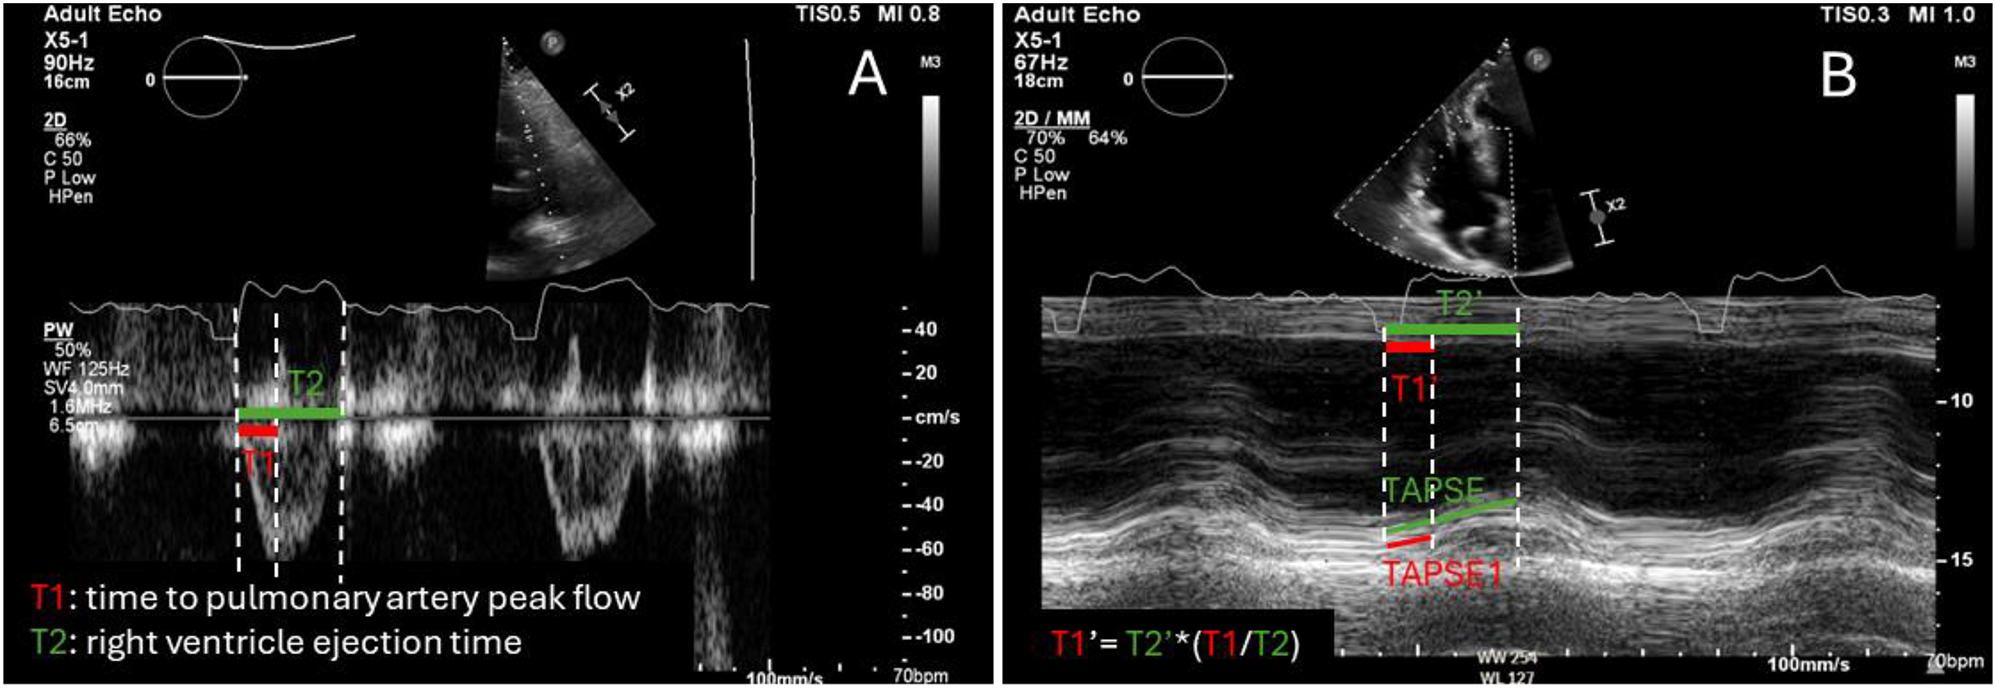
Measurement of first-phase TAPSE (TAPSE1). TAPSE1 was determined on echocardiogram by initially calculating the ratio (T1/T2) between the interval from the onset of systole to peak pulmonary artery flow velocity (T1) and total right ventricular (RV) ejection time (T2), obtained via pulsed-wave Doppler. This ratio was then applied to RV ejection time (T2’) measured separately in the apical four-chamber view to derive the corresponding interval (T1’). TAPSE1 thus represents the tricuspid annular displacement measured from the beginning of systole to the calculated time point (T1’)
